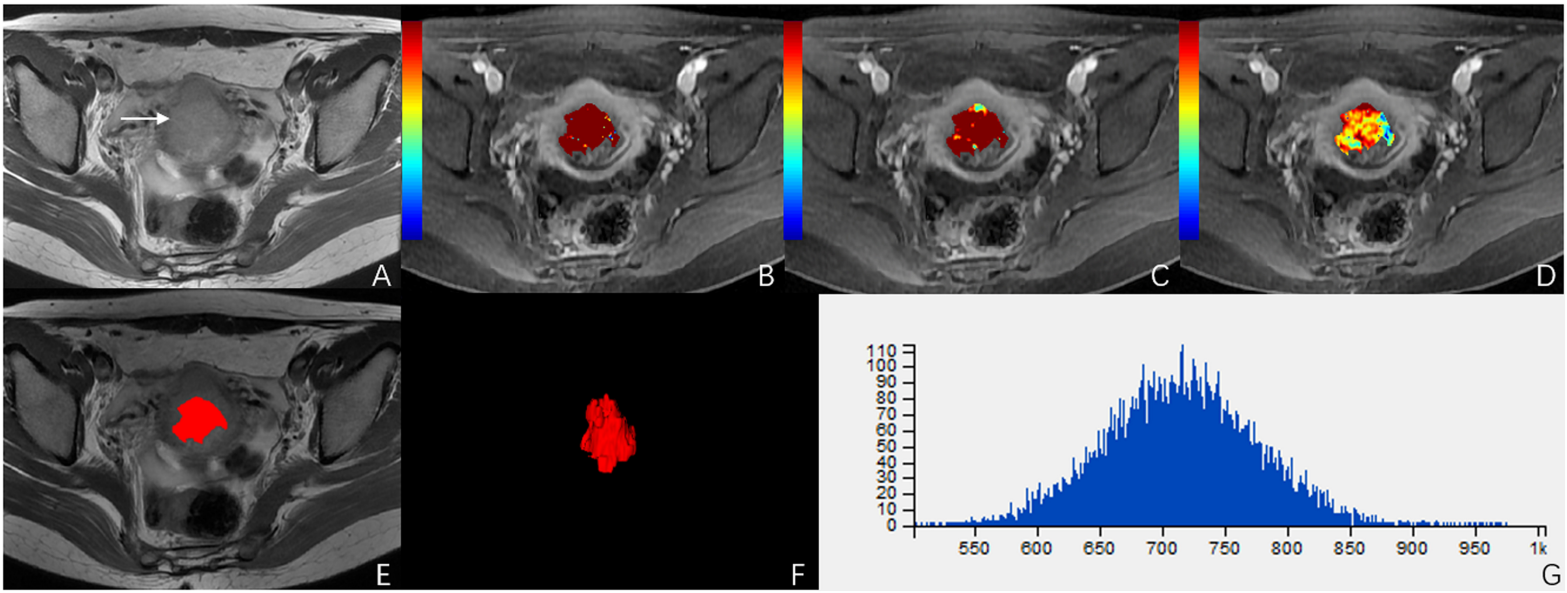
A 51-year-old woman with stage IIB cervical cancer. (A) T2-weighted image (T2WI) shows a slightly hyperintense cervical mass. (B–D) K^trans^, K_ep_, and V_e_ parametric maps are derived from DCE-MRI. The corresponding values are 0.577/min, 0.639/min, 0.694. (E,F) The volume of the tumor is drawn from T2WI. (G) Histogram map of the entire tumor.
